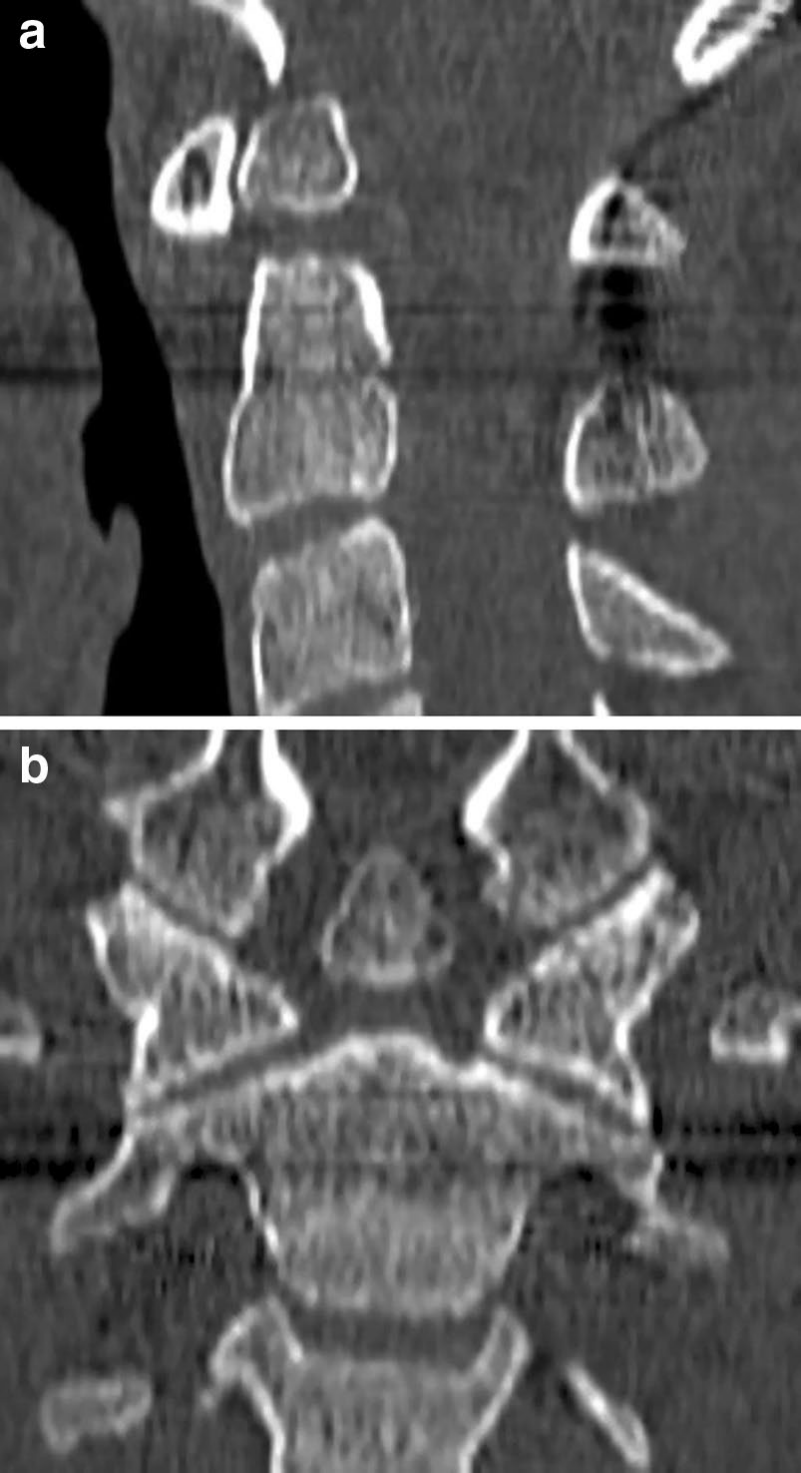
CT example of orthotopic Os Odontoideum. a Sagittal; and b coronal view. Case courtesy of Dr Roberto Schubert, Radiopaedia.org, rID: 14258
